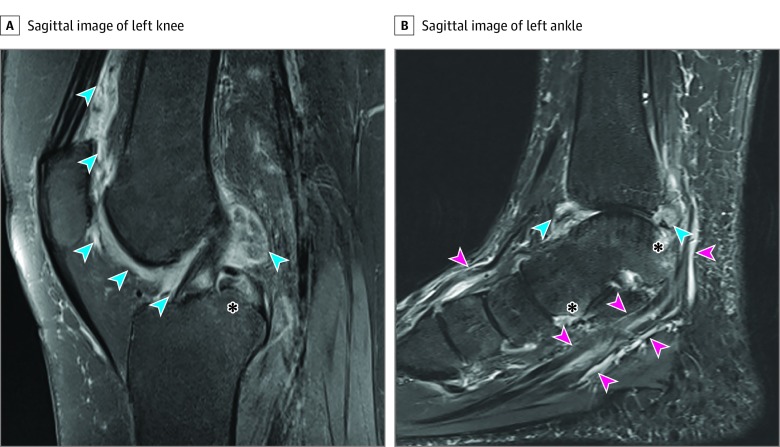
Magnetic Resonance Image of the Knee and Ankle of an Individual With Cervical Cancer and Immune Checkpoint Inhibitor–Induced Inflammatory Arthritis (Patient 7) A, Sagittal fat-suppressed, proton density fast spin-echo image of the left knee depicts extensive, irregular synovial thickening (blue arrows) at the anterior and posterior aspects of the knee and bone marrow edema (asterisk). B, Sagittal image of the left ankle from a short tau inversion recovery sequence demonstrating synovial thickening at the tibiotalar joint (blue arrows), tenosynovitis (pink arrows), and periarticular bone marrow edema (asterisk).
